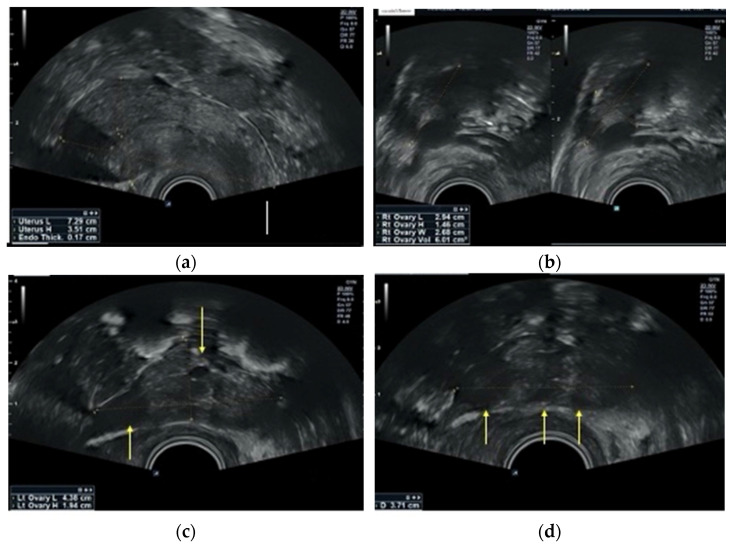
Transvaginal ultrasound on day 3 of the menstrual cycle, 9 months postoperatively. (a) Normal-sized anteverted uterus with thin, early proliferative endometrium. (b) Right ovary of normal size and homogeneous stromal echotexture. (c,d) Left ovary (post-cystectomy) demonstrating a typical “collapsed shell” configuration with tubular morphology and heterogeneous echotexture (alternating hypoechoic and echogenic bands). Yellow arrows indicate small cystic foci within the stromal tissue, possibly representing residual antral follicles within the remodeled ovarian parenchyma.
